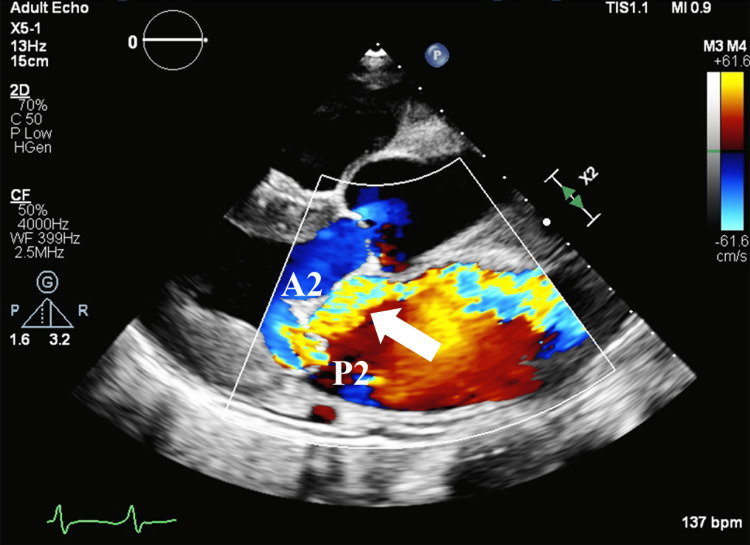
Image of preoperative screening with transthoracic echocardiography There was a mid-posterior mitral leaflet (P2) prolapse with severe MR. The white arrow indicates an MR jet.
